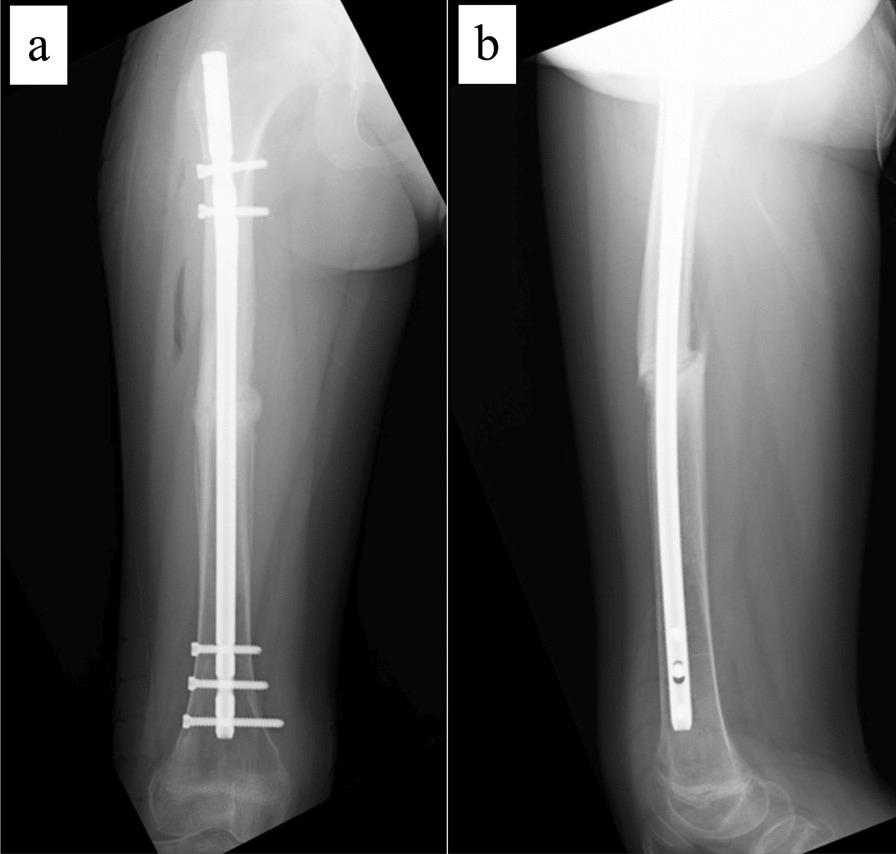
Postoperative radiographs of revision surgery. a Anteroposterior image and b lateral image. The two titanium elastic nails were removed and replaced with a rigid retrograde intramedullary nail. The fracture site was compressed, and the gap is reduced compared with preoperatively
